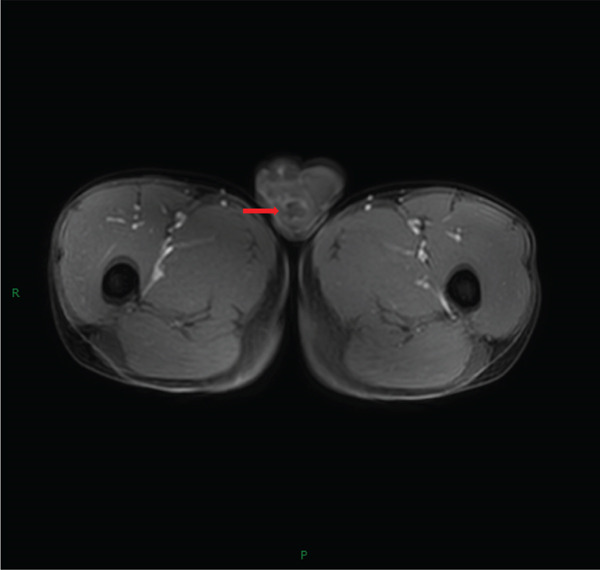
MRI findings of a right testicular mass. Post‐contrast fat‐suppressed T1‐weighted image reveals heterogeneous enhancement (arrow).
